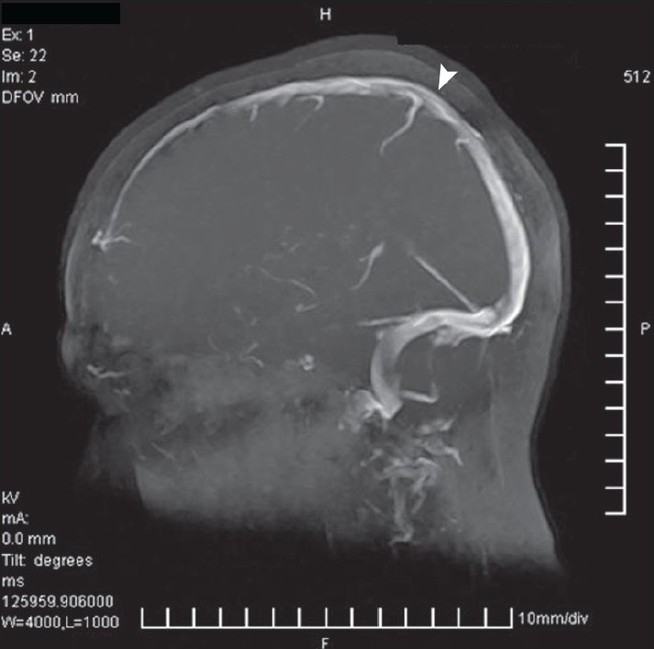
Normal magnetic resonance imaging showing complete filling of venous sinuses without any signal void
